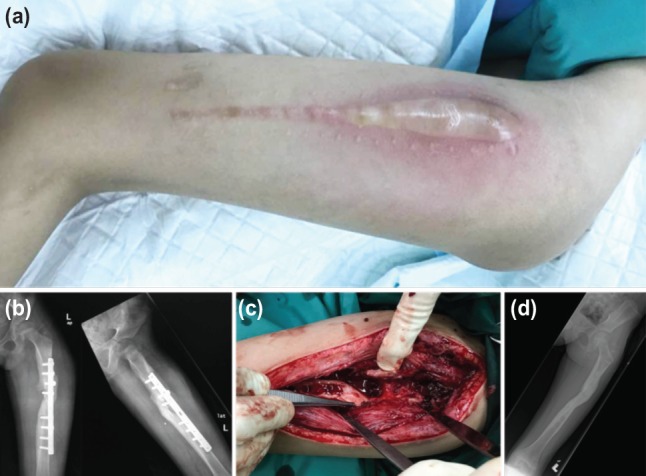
(a) A long surgical scar with a swollen and erythematous left thigh from osteomyelitis of the femur. (b) Radiograph showing osteomyelitis of the left femur and plate loosening. The fracture had united, (c) Intraoperative photograph after debridement and removal of plate. The femur (at the tip of the forceps) had united. (d) Radiograph one year after injury showing fracture remodelling and resolution of infection.
